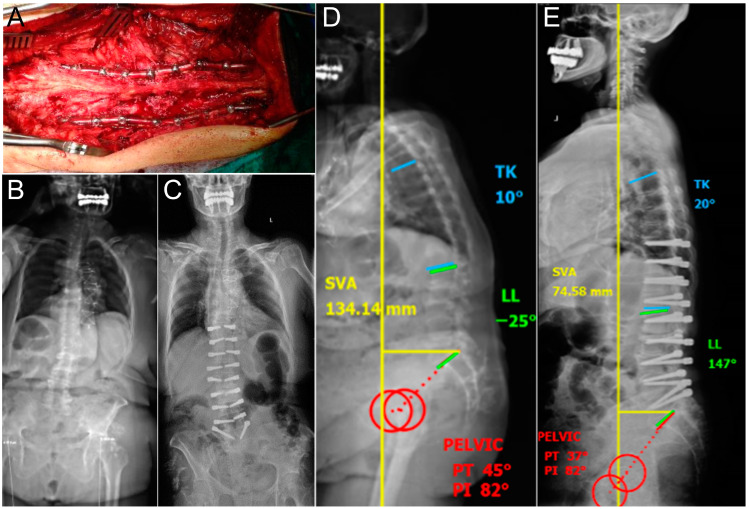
Treatment of kyphosis with Dynesys dynamic stabilization. (A) Intraoperative image showing the posterior stabilization with Dynesys, (B) pre-operative and (C) post-operative coronal dynamic X-rays. (D) Pre-operative and (E) post-operative dynamic sagittal X-rays displaying sagittal parameters of the spine.
